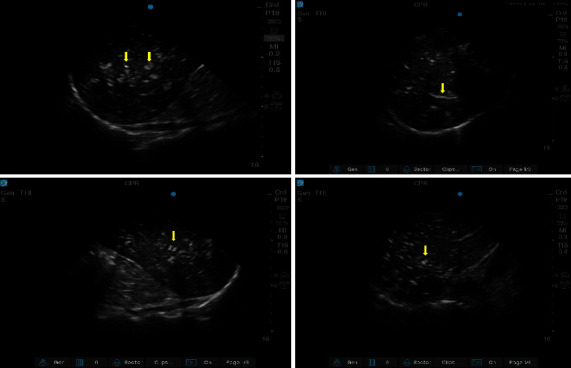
Hepatic portal vein gas observed on ultrasound in traumatic cardiac arrest. The arrow indicated hepatic portal vein gas.
